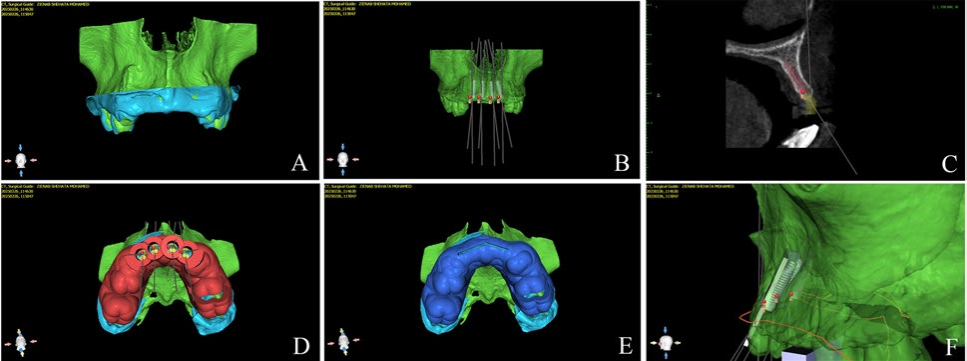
Preoperative digital treatment planning for a ridge splitting case. A CBCT segmentation and superimposition with intraoral scan. B Prosthetically driven implant planning. C Bone width measurement and implant size selection. D Implant placement surgical guide design. E Ridge splitting surgical guide design. F Osteotomy vector determination. CBCT = Cone Beam Computed Tomography
